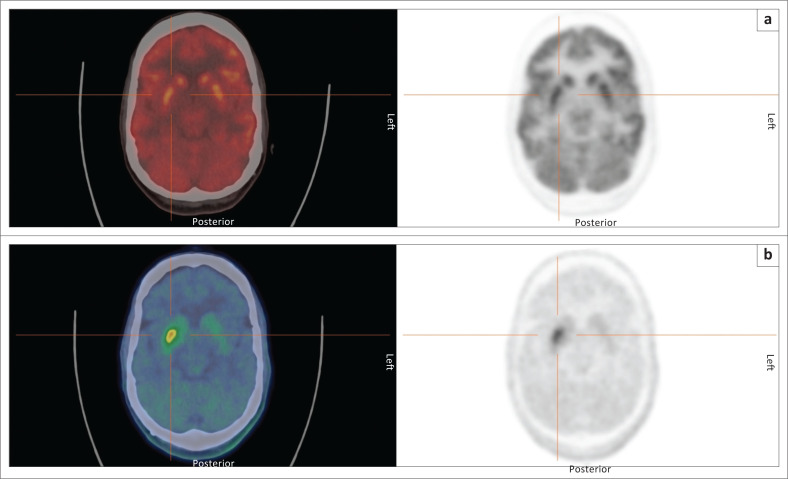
Fluorine-18 FDG (a) and FDOPA (b) PET-CT (fusion) and PET images of a patient with suspected recurrence of a low-grade glioma. The FDG PET-CT study was normal, while FDOPA PET-CT (preferred) demonstrates clear recurrence in the right putamen. This recurrent lesion was initially missed on MRI.
